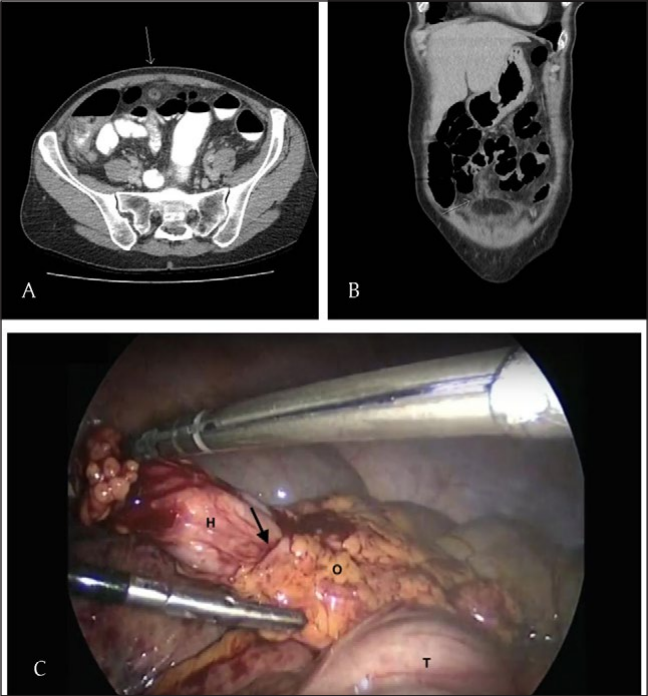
A and B. Axial and coronal CT images of the orifice in the greater omentum containing the pedicle. C. Exploratory laparoscopy shows the herniated pedicle (H) through the defect in the greater omentum (O).
